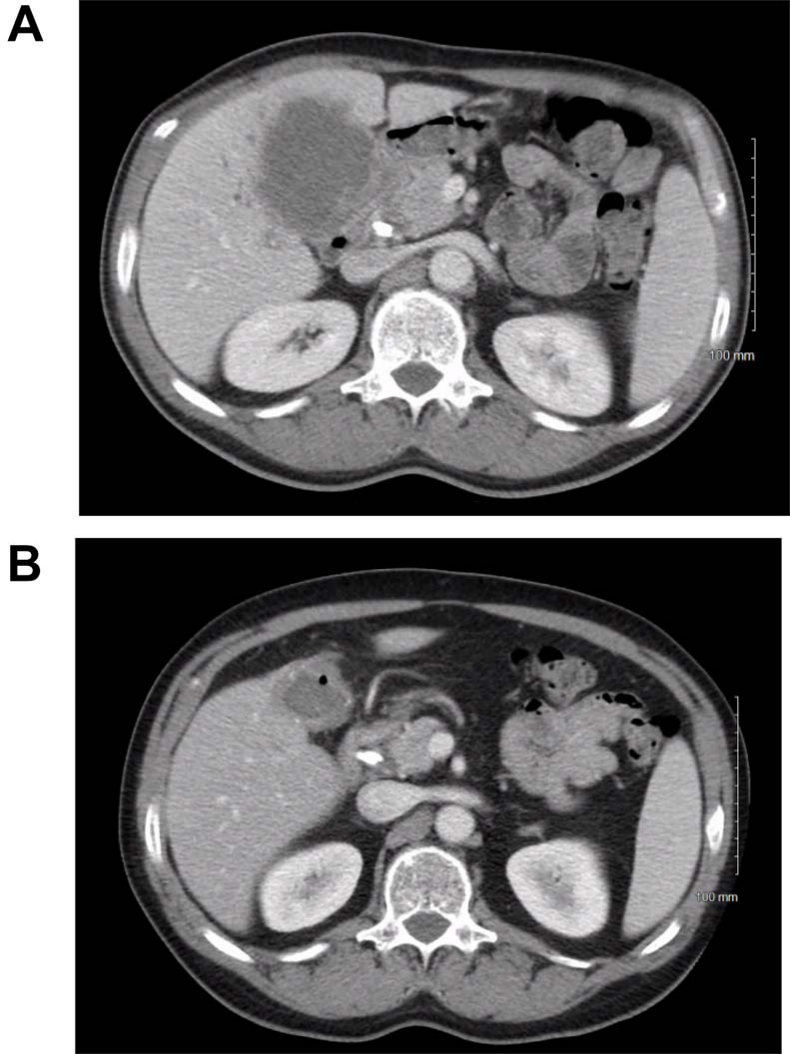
(A) CT scan of the abdomen showing the thickened gallbladder as well as a mass involving hilar hepatic parenchyma; (B) treatment response after immunotherapy.
